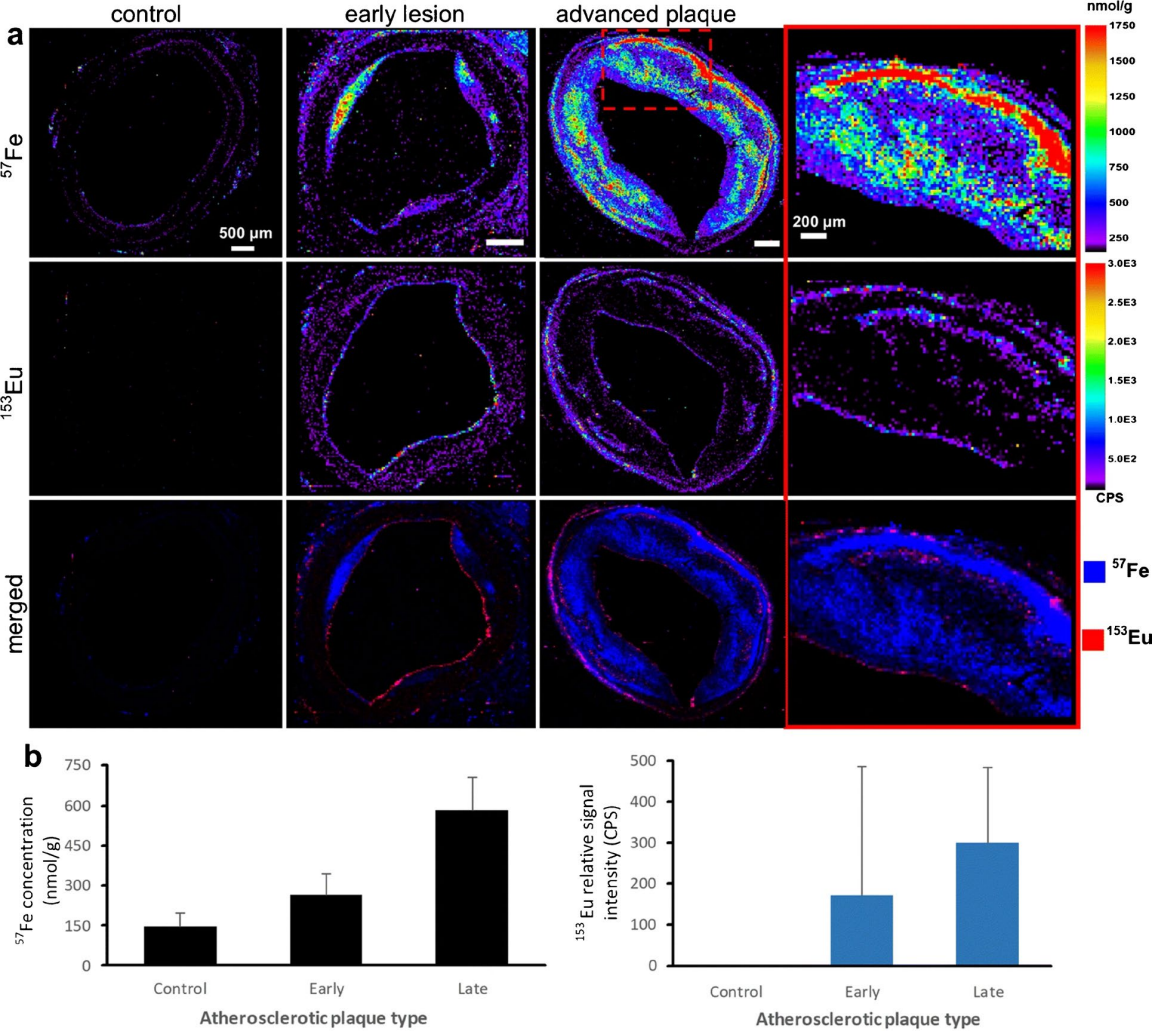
a Eu-doped iron oxide particles and a Gd-based contrast agent were analysed by LA-ICP-MS in control, early, and advanced atherosclerotic plaque progression. Scale bars, 500 µm, ROI, 200 µm. b Comparison of Fe concentrations and Eu signal intensity in atherosclerotic plaques. Reprinted from Springer Nature, Molecular Imaging and Biology, 23, 2021, 382–393, Microdistribution of magnetic resonance imaging contrast agents in atherosclerotic plaques determined by LA-ICP-MS and SR-μXRF imaging, Y.O. Uca, D. Hallmann, B. Hesse, C. Seim, N. Stolzenburg, H. Pietsch, J. Schnorr, M. Taupitz [46]. Copyright (2020), Uca et al.
